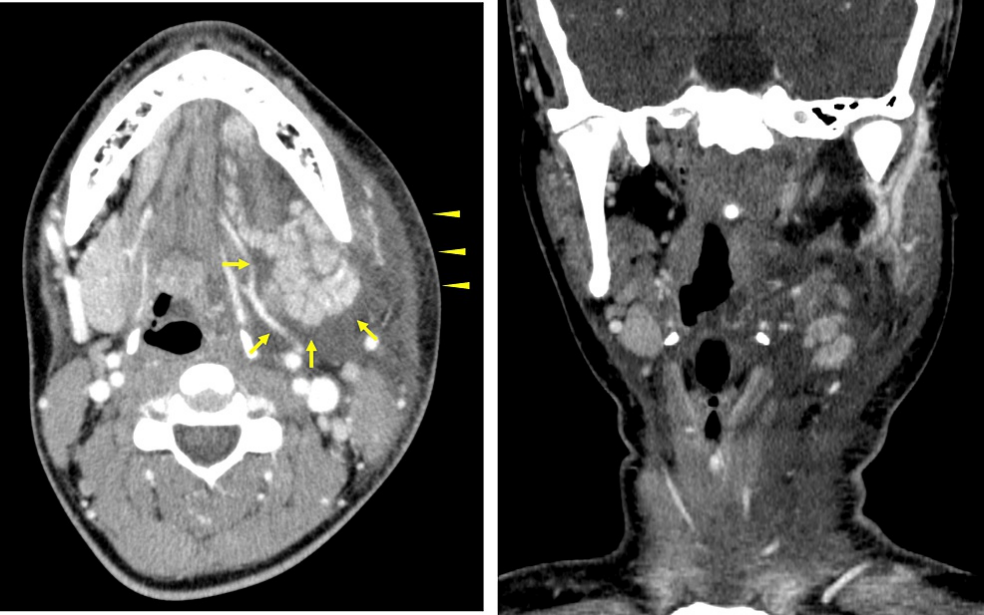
Contrast-enhanced neck CT images (axial and coronal views) Swelling and lobulation of the left submandibular gland with surrounding fluid density (arrow) and increased density of the cervical subcutaneous adipose tissue (arrowhead) are observed. CT: computed tomography
